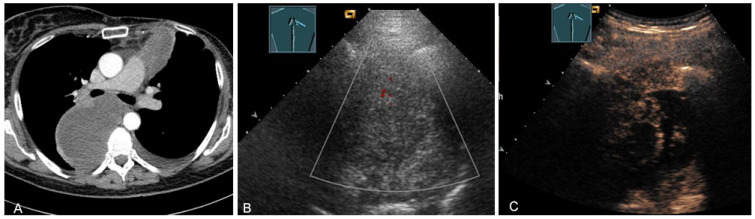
A patient with tumor formation in the anterior and paravertebral mediastinum on CT (provided by Prof. Dr. Andreas H. Mahnken, Marburg, Germany) (A), B-mode US (B), and CEUS (C), with anamnestic phyllodes tumor of the breast, confirmed by biopsy as mediastinal metastasis.
